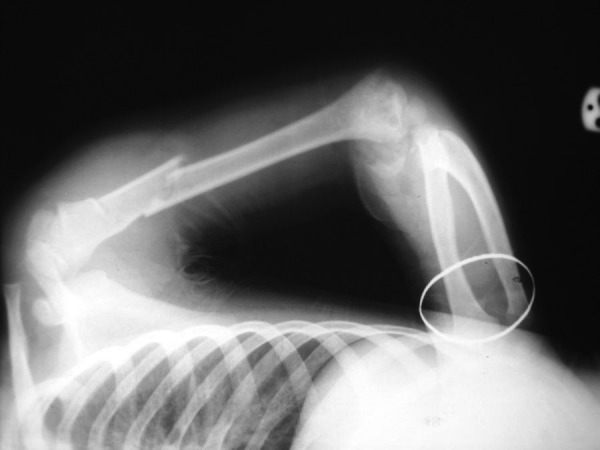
Initial X-Ray of the Fracture
